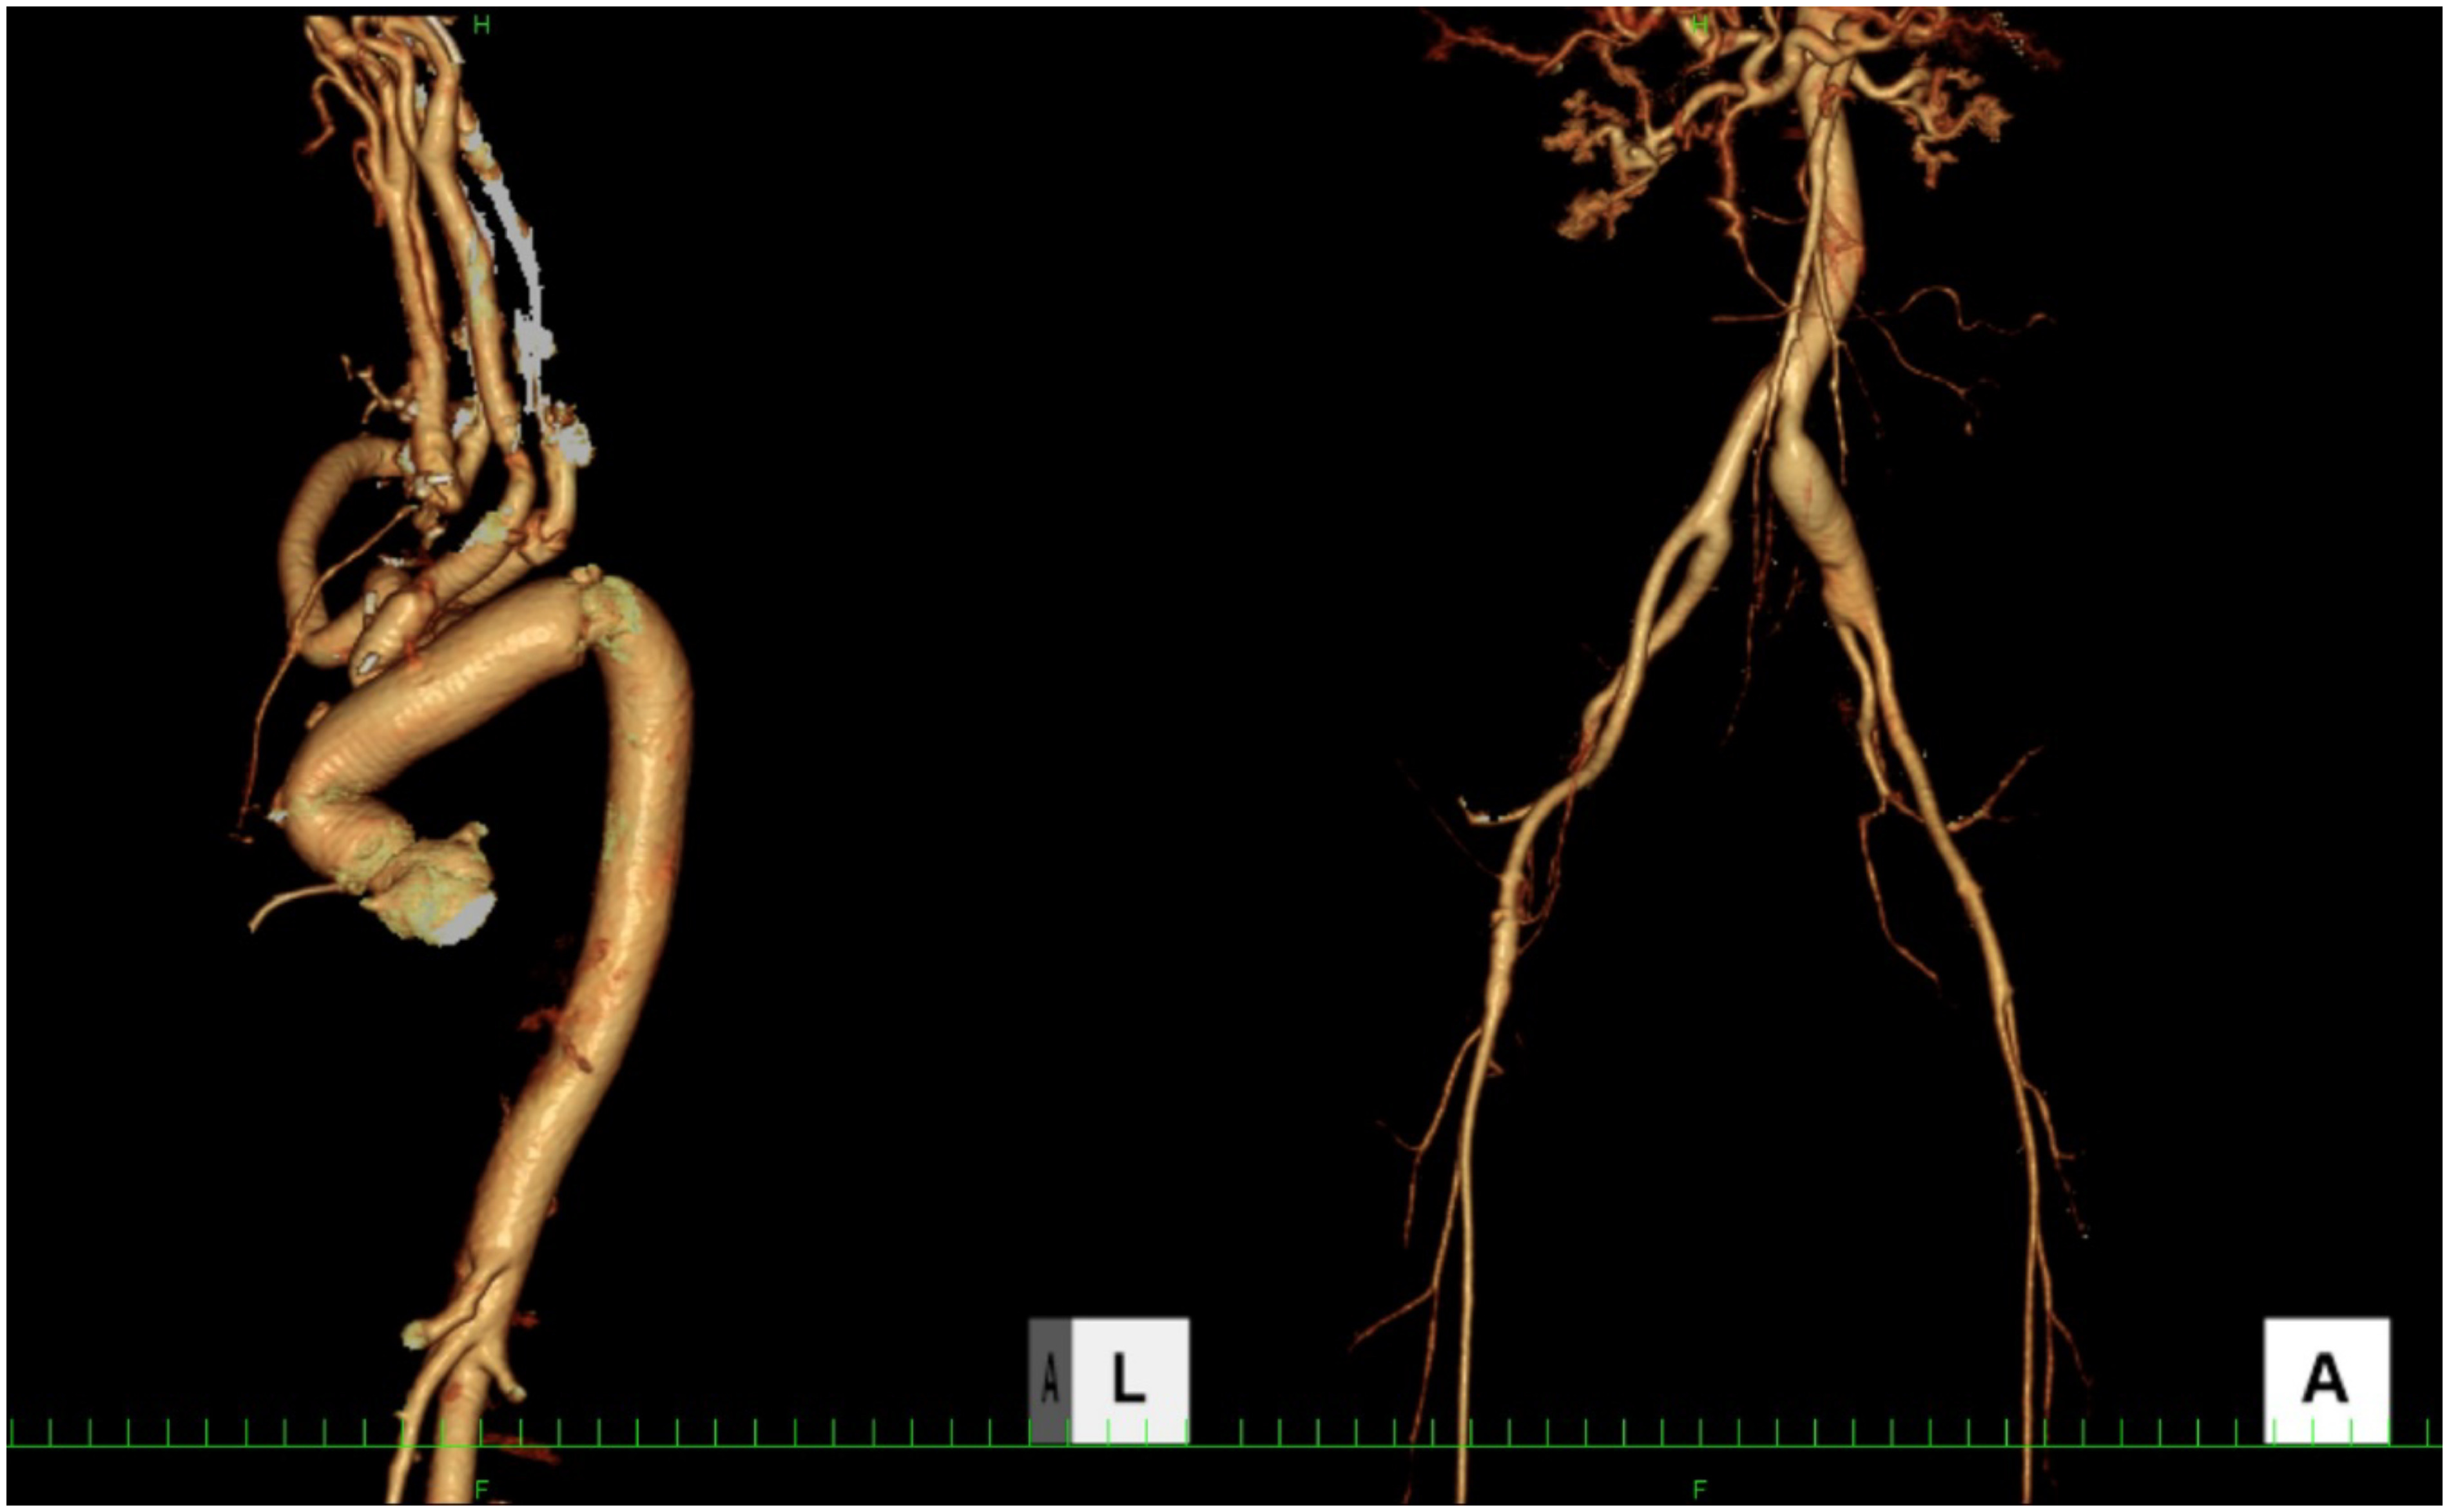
Fig. 2 Three-dimensional reconstruction of a postoperative computed tomography scan showing complete thrombosis of the false lumen without abnormal findings of anastomotic site and new aortic aneurysm or dissection.
